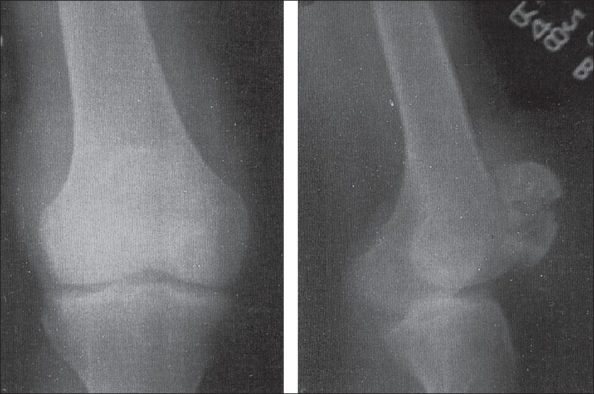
X-ray anteroposterior (AP) and lateral view of knee joint shows a transverse fracture of patella with a lytic lesion occupying most of the two halves of the patella. There was no periosteal reaction. A thin sclerotic rim was present with fine matrix calcification with in the lesion
